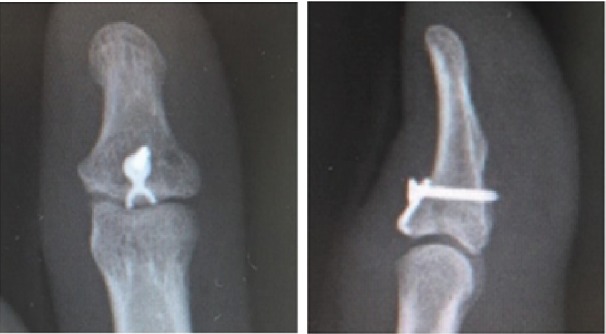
The radiograph images after reduction and fixation.
